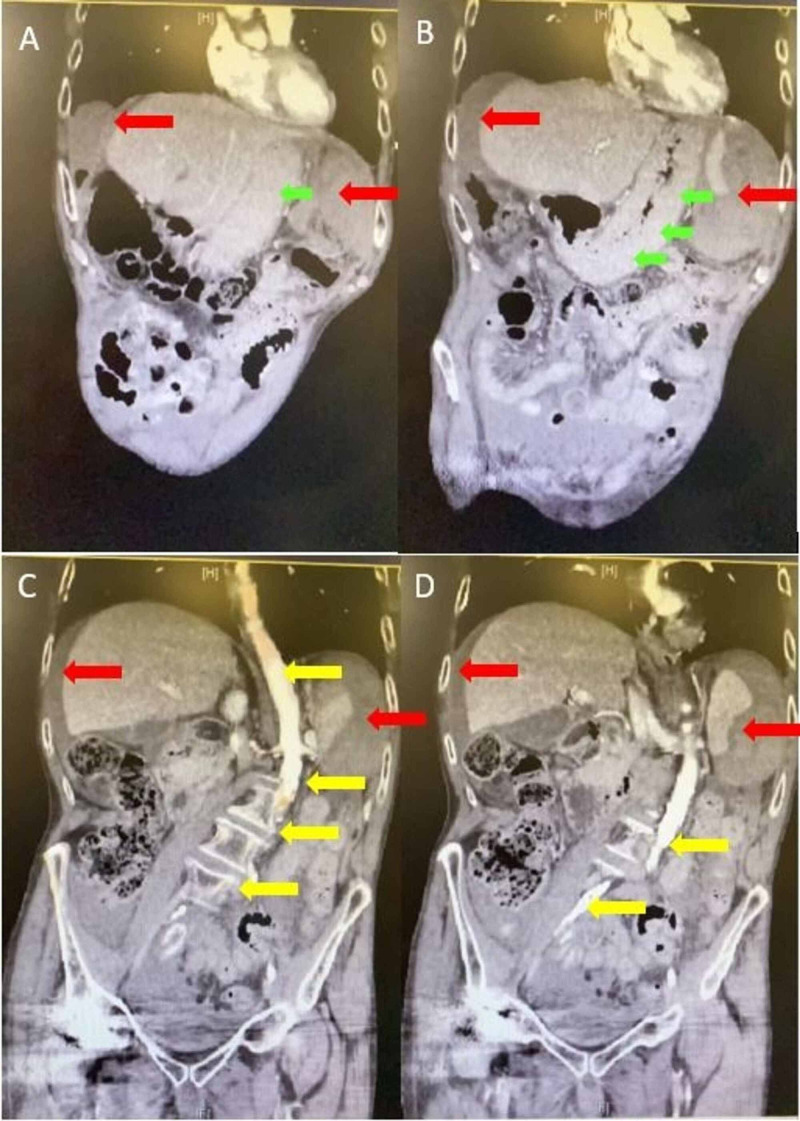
Series of four coronal images from abdominal CT. Splenic injury with hemoperitoneum (A-D, red arrows), scoliosis/angulated aorta (C and D, yellow arrows), stomach with severe wall thickening (A and B, green arrows). CT, computed tomography
